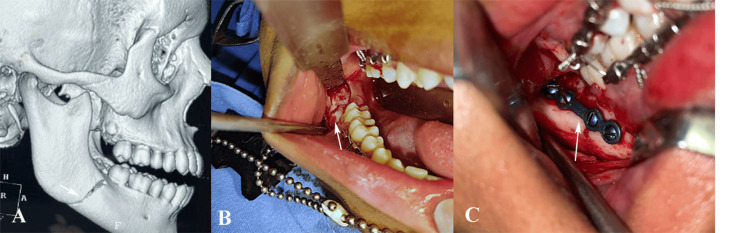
Transoral approach for open reduction and internal fixation (ORIF) of mandibular angle fracture, (A) Cone-beam computed tomographic scan showing unilateral mandibular angle fracture, (B) Intraoral exposure of the mandibular angle fracture site, (C) Fixation of the fracture using 4-hole miniplates and screws via intraoral access. Original clinical images of a patient from the study, used with the patient’s informed consent.
